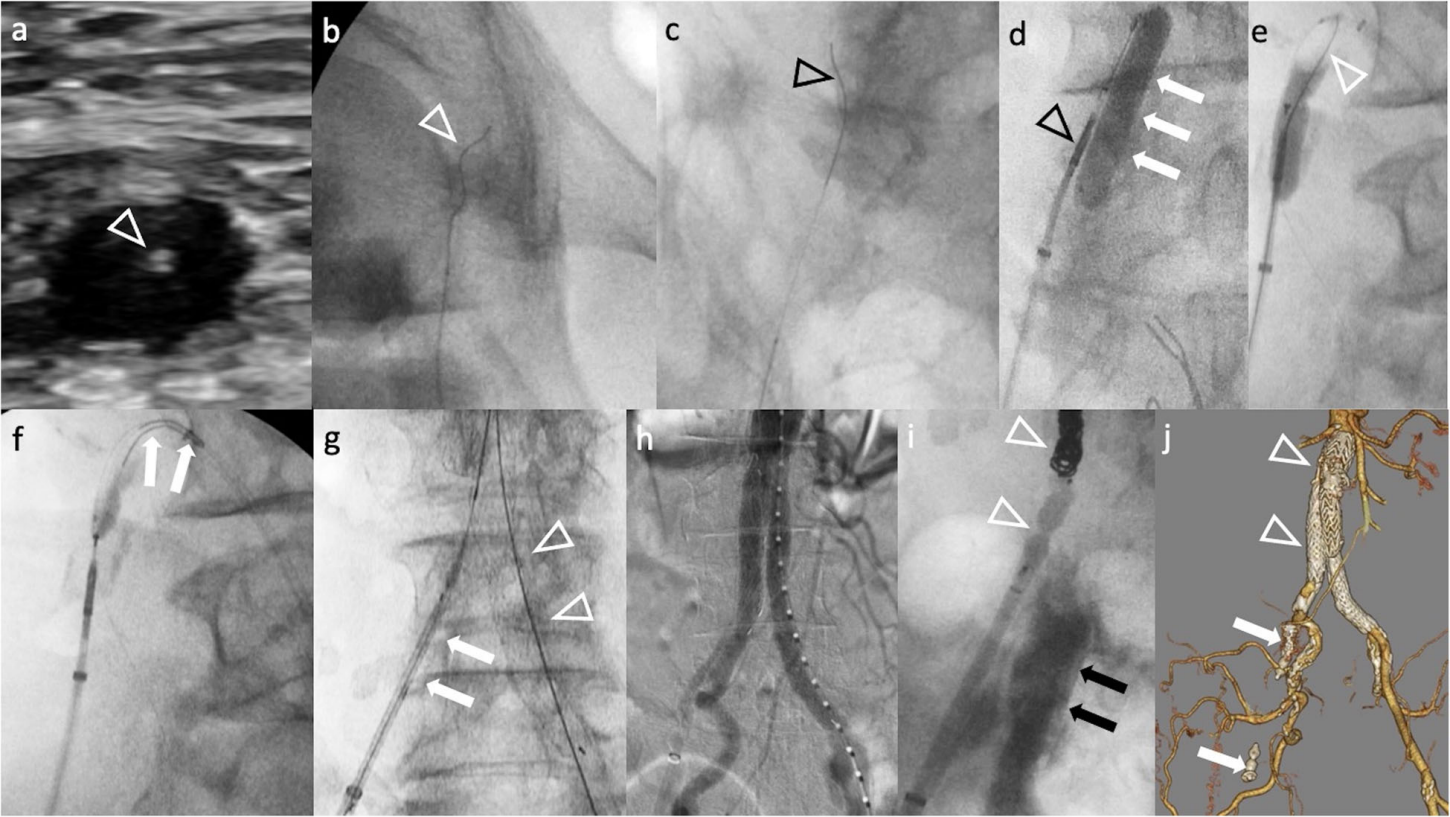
Procedure detail. a The occluded right CFA was punctured under ultrasound guidance (arrowhead: tip of the needle), b A guidewire (arrow heads) was advanced via the occluded right CFA, c A guidewire was advanced to the subintimal space of the right CIA, d The OUTBACK reentry device in the subintimal space (arrow head) was directed to an angioplasty balloon located at the lumen of the right CIA (arrows), e Insertion of the wire (arrowhead) into the punctured balloon, f Retracting the punctured balloon with the wire established a through and through wire, g Main body graft (arrowheads) which was delivered via left iliac artery was deployed and then the contralateral limb (arrows) was delivered via right iliac artery, h Completion angiogram shows a successful exclusion of the AAA, i Embolization of the tract was performed using coils and a plug (arrowheads) where a 12F sheath was advanced. A leakage of contrast media (arrows) indicates vessel disruption, j Post-operative CT shows implanted endograft (arrowheads), coils and plugs (arrows), and preserved collateral channels. AAA: abdominal aortic aneurysm, CFA: common femoral artery, CIA: common iliac artery
